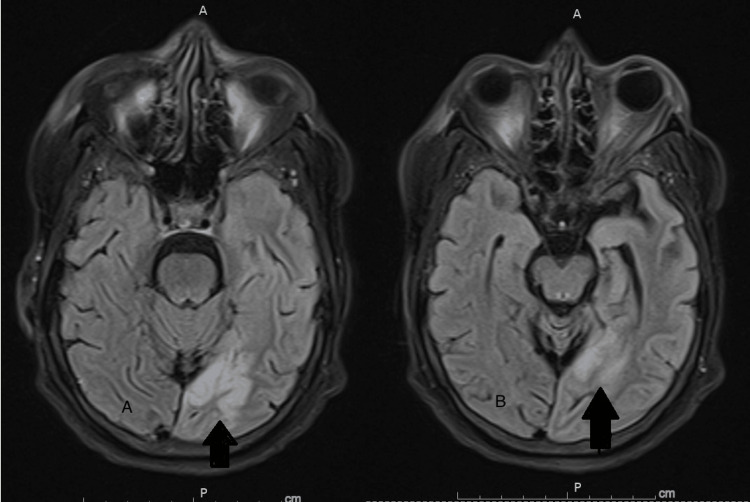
Further delineation of fluid attenuation inversion recovery (FLAIR) changes on MRI brain MRI brain showing significant hyper-intensity in FLAIR sequence affecting the left posterior parietal and occipital lobes (arrows in A and B).
